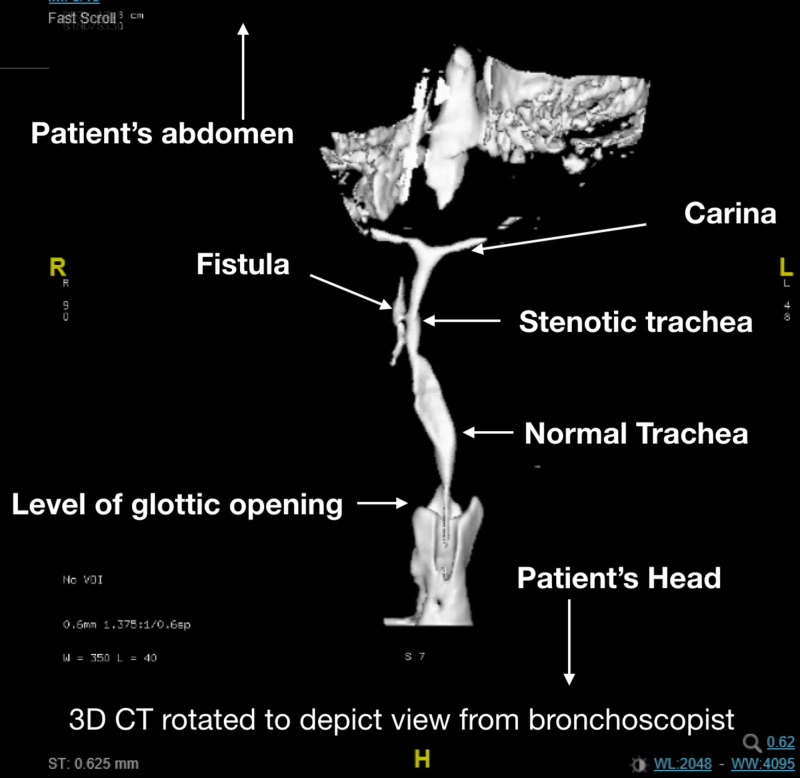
Enhanced 3D reconstruction and subtraction of the trachea. Three-dimensional CT airway reconstruction rotated to depict the anatomy on bronchoscopy in a patient in the supine position.
